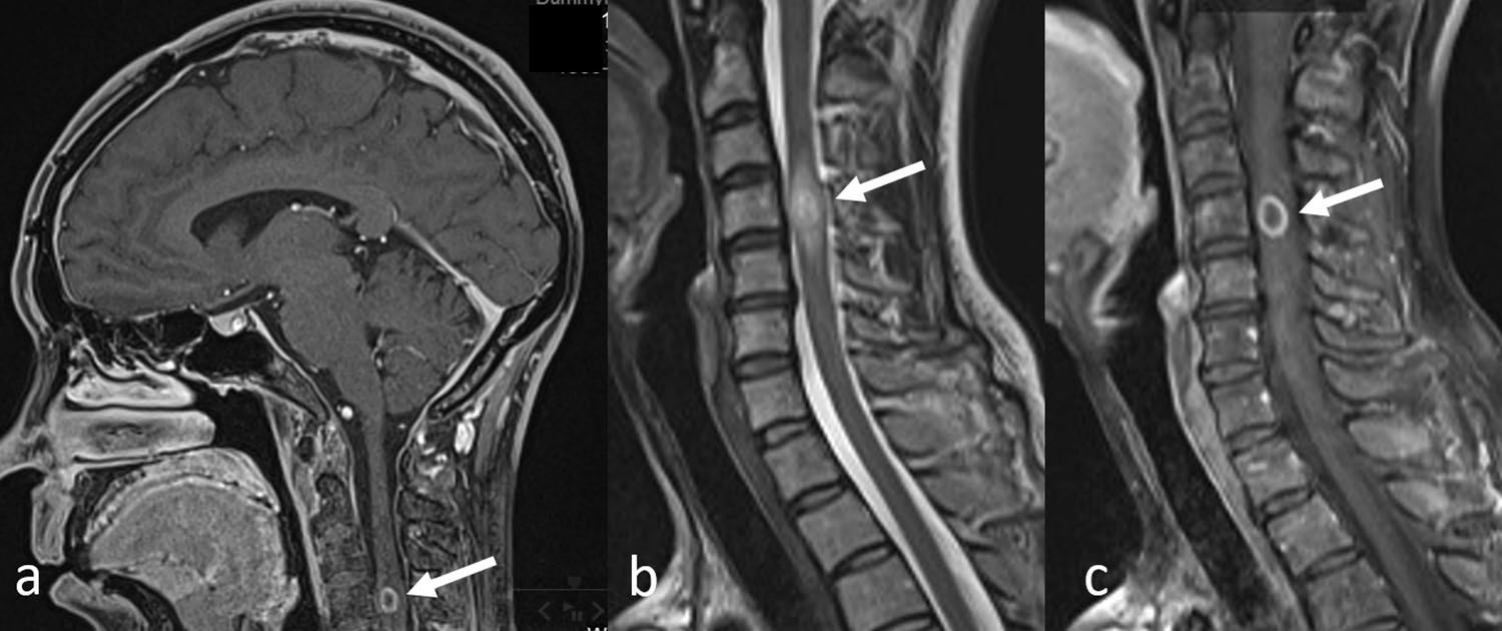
Intramedullary spinal cord metastasis in a patient with lung adenocarcinoma. Contrast-enhanced 3D T1-weighted head MR image in the sagittal view a shows a ring-enhanced intramedullary tumor (arrow). Sagittal T2-weighted b and contrast-enhanced T1-weighted c spine MR images show characteristic findings of extensive edema surrounding the tumor (arrows). *MR* magnetic resonance
